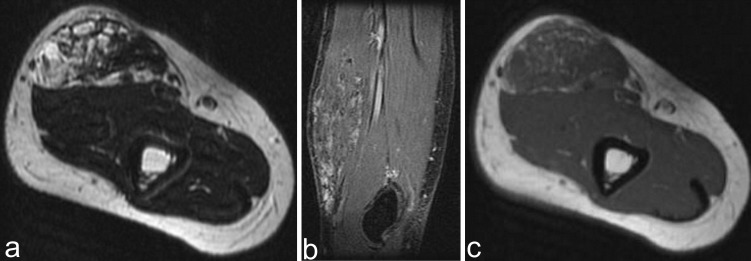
1.5T MRI showing lobulated and septated tumor in the biceps brachial muscles, with high signal intensity on T2 weighted and fat suppression images with calcification (a,b); intermediate signal on T1 weighted images (c)
